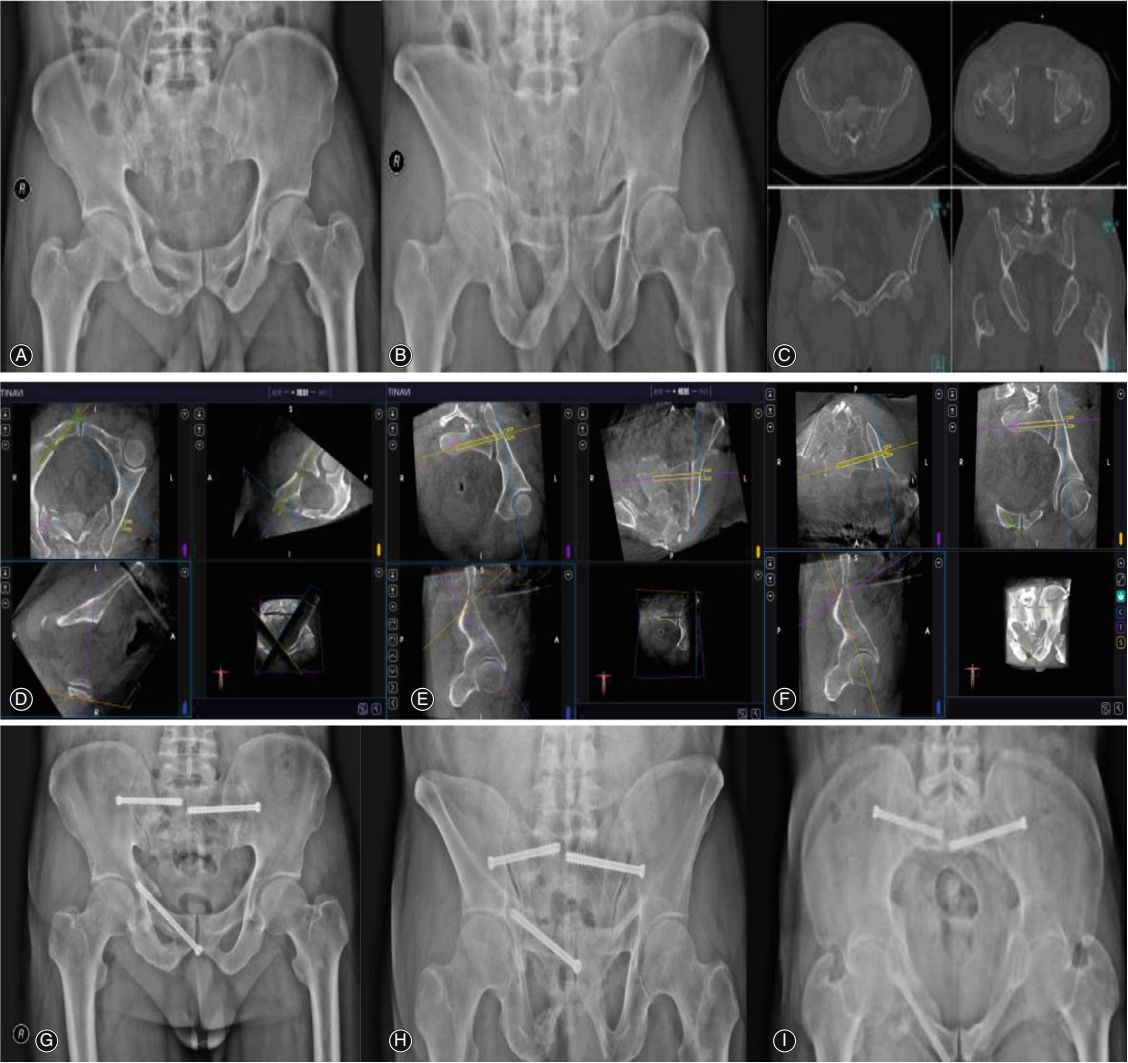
A 67‐year‐old man with a fragility fracture of the pelvis (FFP IVc type). Preoperative, and intraoperative planning and postoperative images of TiRobot‐assisted channel screw fixation. (A,B) Preoperative anteroposterior (AP) and outlet radiographs of the pelvis show the bilateral sacroiliac joint separation and the right acetabular anterior column fracture. (C) Horizontal and coronal CT of the pelvis showed a “close‐book” injury of the right sacroiliac joint, a “crescent” fracture of the right posterior iliac bone, a compression fracture of the Dennis II region of the right sacrum, a partially overlapping and displaced fracture of the right superior pubic branch (anterior acetabular column) and subpubic branch, an “open‐book” manifestation of the left sacroiliac joint, and a non‐displaced fracture of the left superior pubic branch (anterior acetabular column). (D–F) Intraoperative planning images. (G–I) Postoperatively, anteroposterior (AP), outlet, and inlet radiographs of the pelvis showed good reduction and internal fixation. Bilateral sacroiliac joint screws and right anterograde anterior column screws were in good position.
